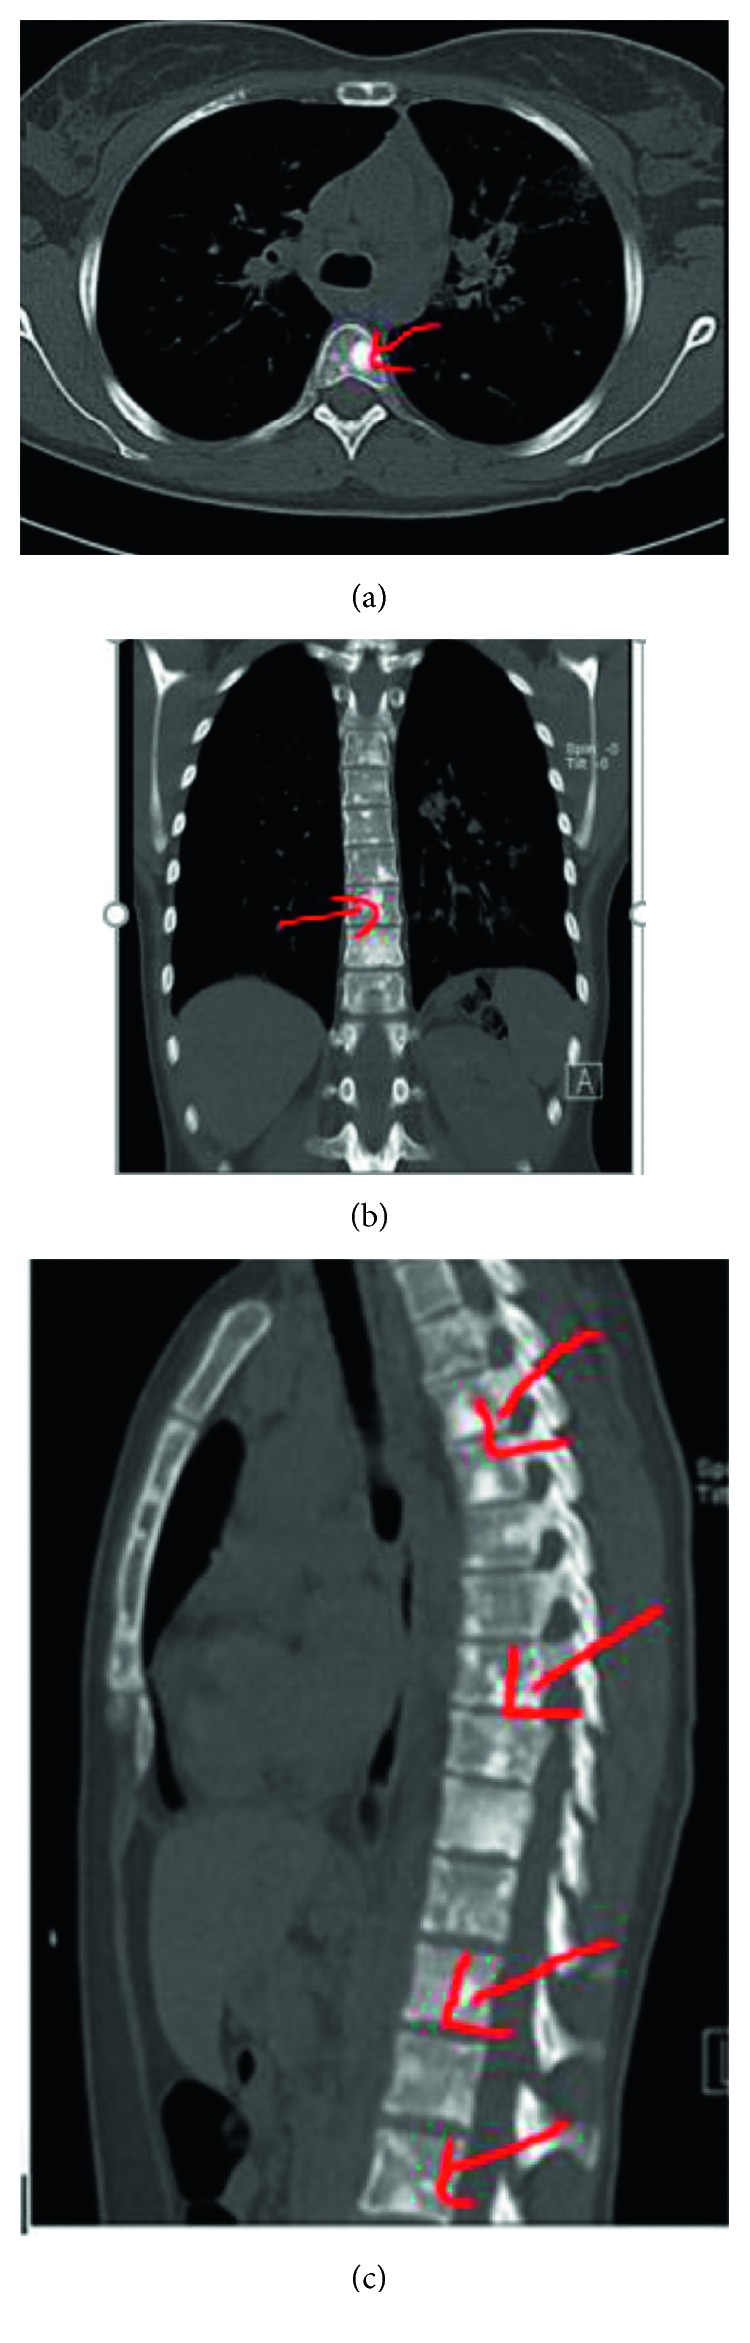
CT bone window showing sclerotic bone metastases.
